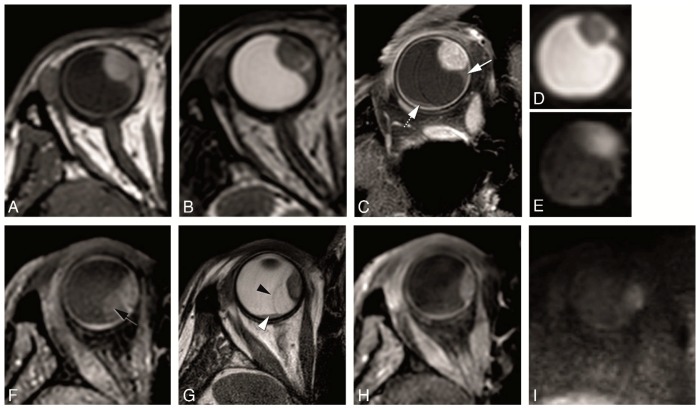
(A–I) Retinal detachment in two different patients. (A–E) MS 1 mm axial T1 (A), MS 1 mm axial T2 (B), MS 2 mm enhanced axial oblique T1 with fat signal suppression (C), DWI with b values of 0 s/mm^2^ (D) and 800 s/mm^2^ (E). Small homogeneous retinal detachment (arrow) located adjacent to the tumor, but also posterior and temporal (dashed arrow). It is distinguished from the tumor due to no enhancement, no diffusion restriction and a lentiform shape. (F–I) MS 1 mm axial T1 with fat signal suppression (F), MS 2 mm axial T2 (G), MS 1 mm enhanced axial T1 with fat signal suppression (H) and DWI with b value of 800 s/mm^2^ (I). Large heterogeneous retinal detachment. One bigger component which is better seen on T1 without contrast (black arrow), while on T2 only the retina being seen (black arrowhead) and the content being similar to the vitreous. One smaller component better seen on T2 (arrowhead), and due to being hemorrhagic hyperintense on T1 and with slight diffusion restriction.
